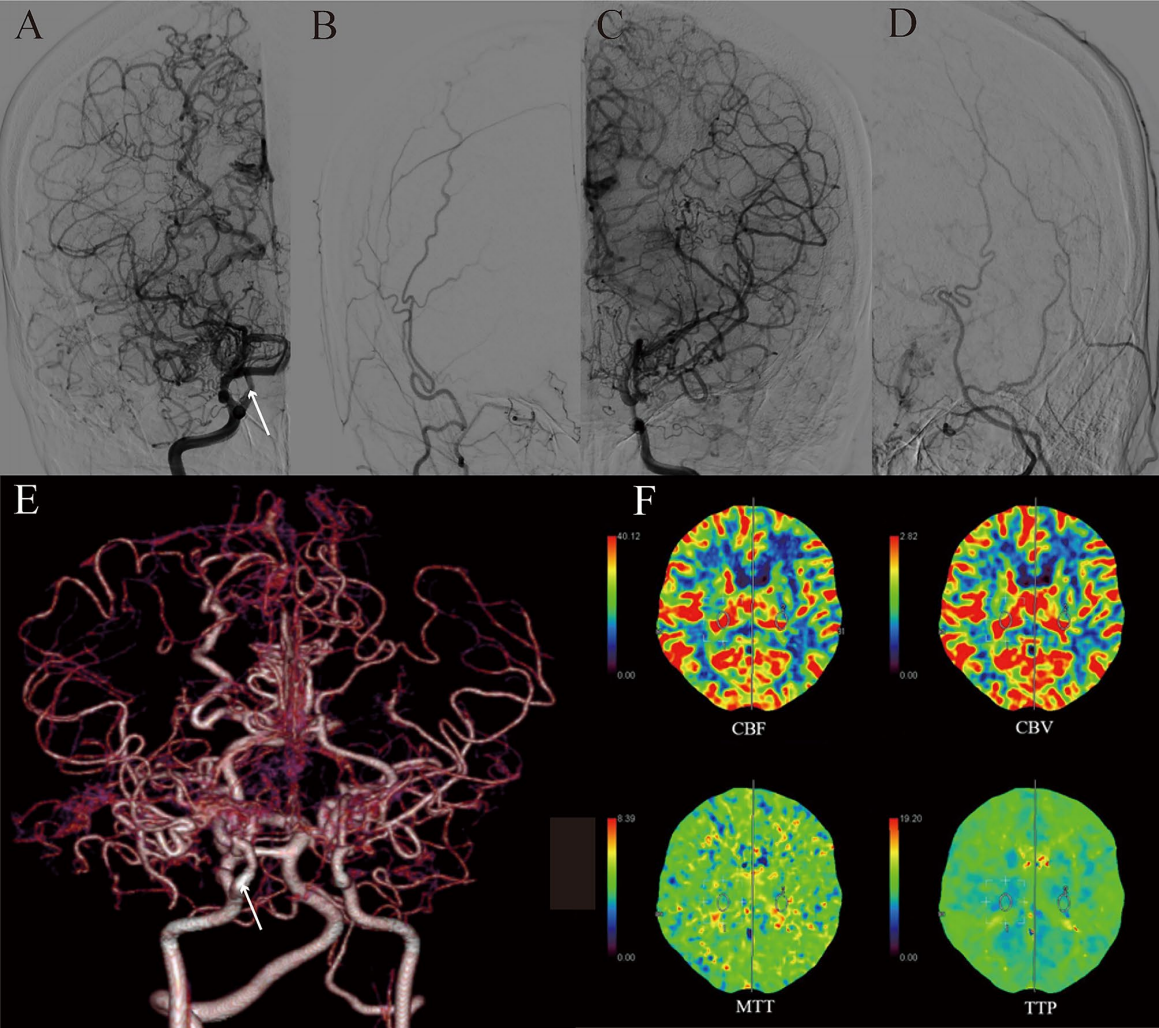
First admission CTP imaging showed old infarction and partial liquefaction in the left frontal and temporal lobe (F), and the CTA and DSA revealed the PTA arises from cavernous portion of the right ICA (A, E) (white arrow), and stenosis or occlusion in the bilateral ACAs, the M1 segments of bilateral MCAs, and the C5-C6 segments of bilateal ICAs, but the anterior and posterior communicating arteries were patent (A, B, C, D, E). Moreover, there were a great mount of moyamoya vessels (A, B, C, D, E). ICA: internal carotid artery; CTP: computed tomography perfusion; CTA: computed tomography angiography; DSA: digital subtraction angiography. ACA: anterior cerebral artery; MCA: middle cerebral artery; PTA: persistent trigeminal artery
